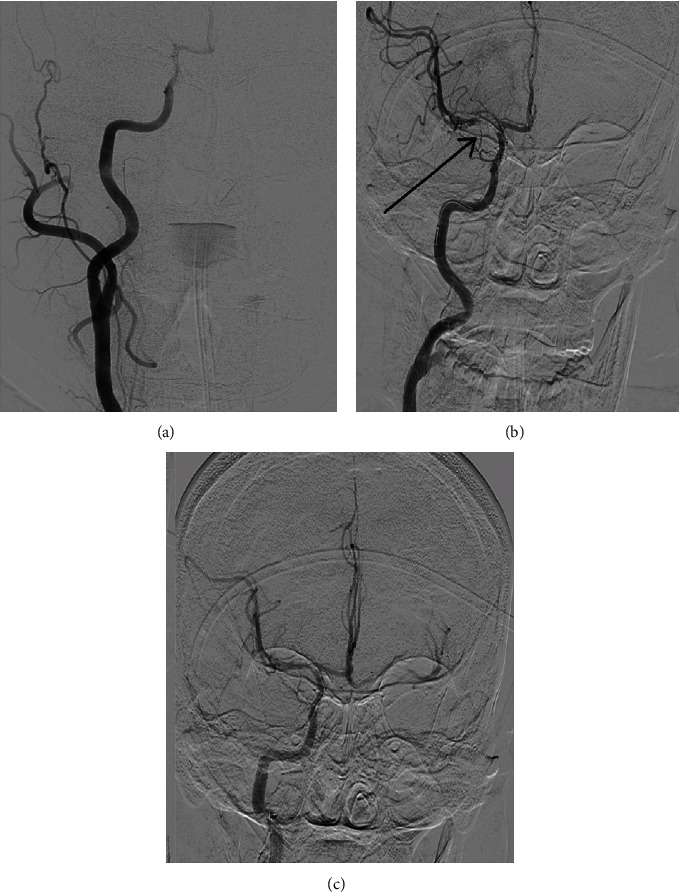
CT cerebral angiogram shows (a) distal ICA occlusion with non-visualization of M1 MCA, (b) stentriever in situ with left ICA run showing a filling defect in distal ICA proximal M1 MCA (arrow), and (c) complete recanalization of ICA MCA.
